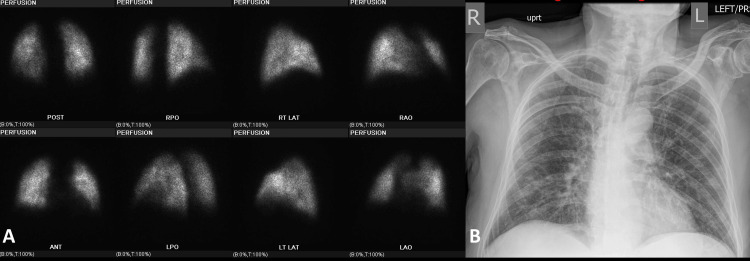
Low probability for PE in a 76-year-old male positive with COVID-19 (A) Images from lung perfusion scintigraphy performed with 99mTc-MAA, near homogeneous tracer distribution in both lungs. No distinct segmental perfusion defect noted. Based on the modified PIOPED II perfusion-only criteria, the study is best classified as a low-probability scan for pulmonary embolism. (B) A chest radiograph shows patchy ground-glass opacities of both lungs in a peripheral distribution in a known case of COVID-19.
